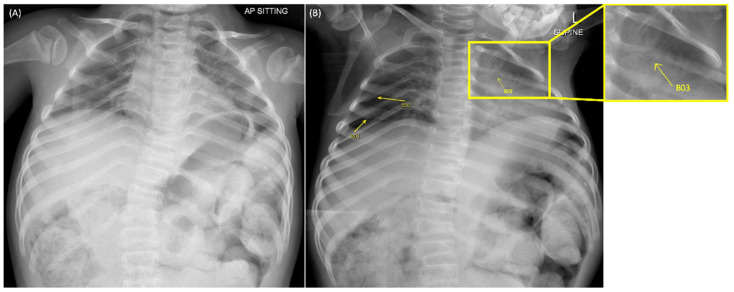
Chest radiographs of the patient; anterior−posterior chest radiograph (A) and left oblique chest radiograph (B). A right anterior fourth rib healing fracture with callus formation (B01) and a new right posterior fourth rib fracture (B02) were reported by the radiologists. There was a concern from the attending emergency physician about an unreported new fracture at the left posterior third rib (B03; enlarged view in yellow box).
